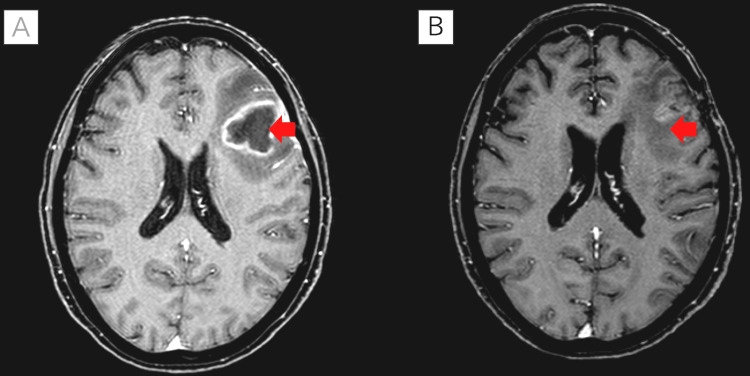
MRI of brain abscess formation and post-treatment changes (red arrows) (A) This MRI scan shows the formation of a brain abscess, indicated by a ring-enhancing lesion. (B) This MRI scan shows the area after the operation for the brain abscess, highlighting the resulting scarring.
